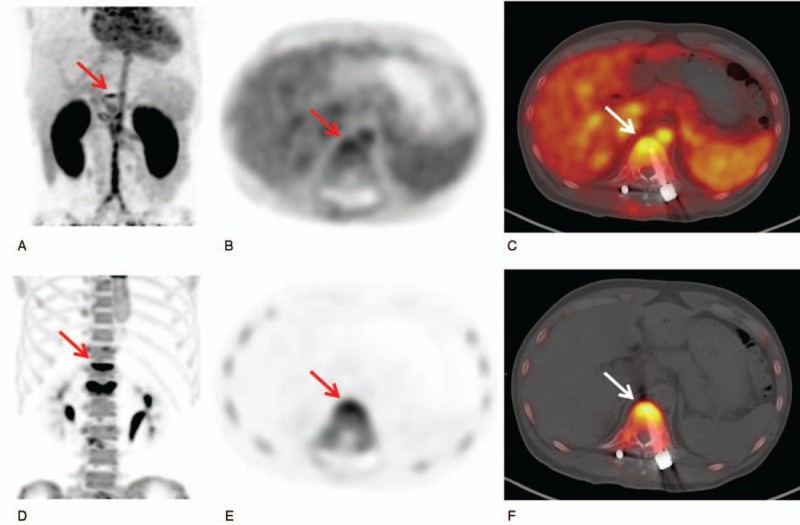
A maximal intensity projection image (A) and transaxial PET (B) and fused PET/CT (C) images from the early-phase scan and a maximal intensity projection image (D) and transaxial PET (E) and fused PET/CT (F) images from the bone-phase scan in F-18 NaF bone PET/CT of a 34-year-old man. Both early-phase and bone-phase scan images showed focally increased radiotracer uptake at the surgical site of the T12 spine (arrows in A–F). The patient underwent a revision operation and was diagnosed with surgical site infection of the spine. PET/CT = positron emission tomography/computed tomography.
